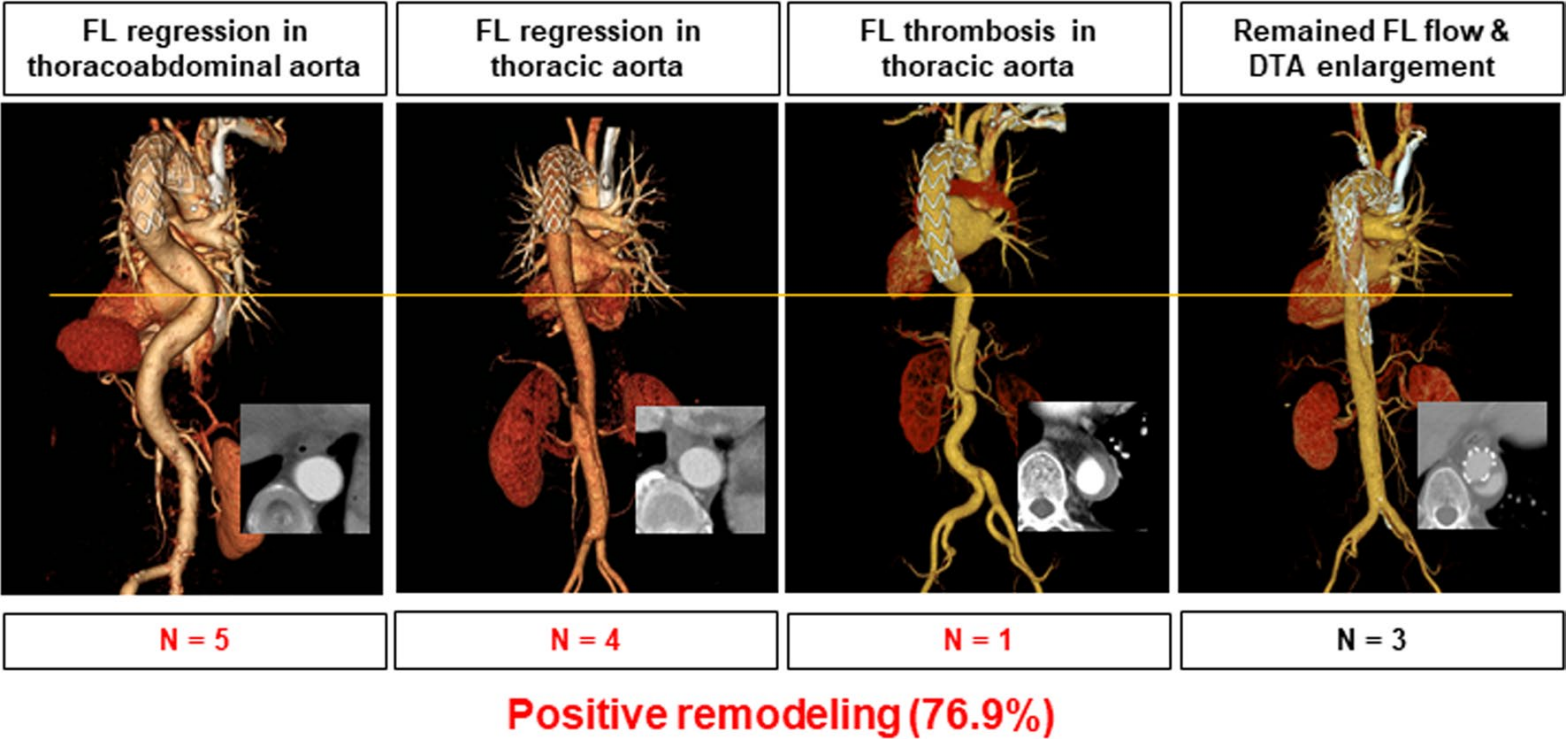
Aortic measurements after zone 2 hybrid thoracic endovascular aortic repair for acute type B aortic dissection. The transverse line on the figure points the distal descending thoracic aorta level. The same level of CT axial images is presented together. False lumen regression over the entire thoracoabdominal aorta was observed in five patients (38.5%), false lumen regression at the thoracic aorta was observed in four patients (30.8%), partial false lumen thrombosis of the thoracic aorta was found in one patient (7.7%), and remaining false lumen flow or enlargement of the descending thoracic aorta was noted in three patients (23.0%). The rate of positive aortic remodeling was 76.9%. FL, false lumen; DTA, descending thoracic aorta
